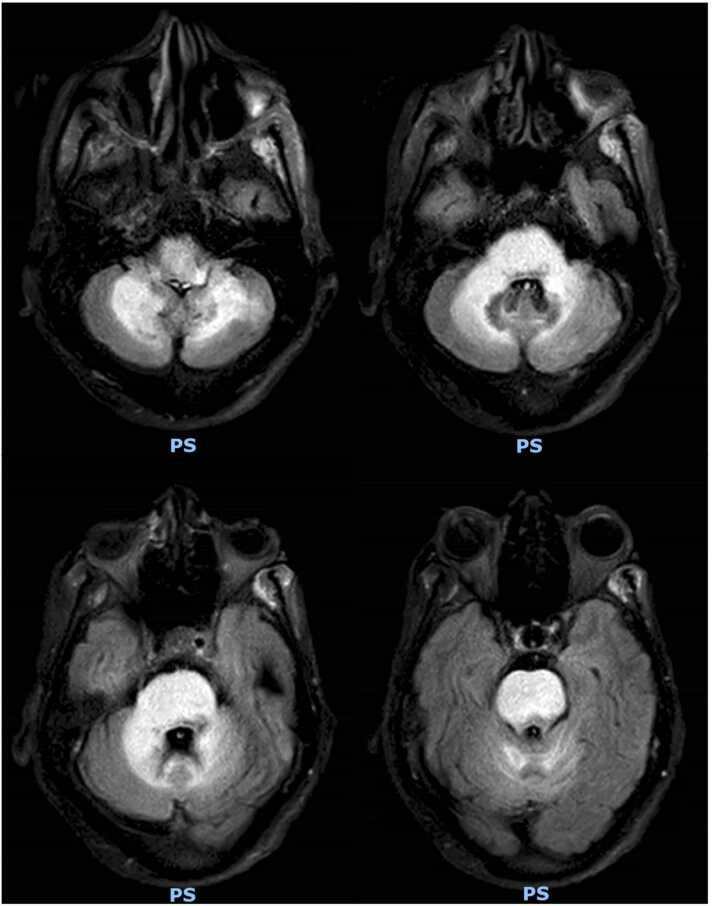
MRI Brain with and without contrast; axial T2 FLAIR (May 28th, 2022). Significant interval worsening of signal abnormalities in the posterior fossa with now diffuse involvement of the pons, extension to the mesencephalon and markedly more pronounced involvement of the medulla, right middle cerebellar peduncle, and central cerebellum. Patchy areas of restricted diffusion are without a clear vascular territorial distribution. IRIS is less likely in the absence of contrast enhancement, but it cannot be completely excluded in the appropriate scenario.
